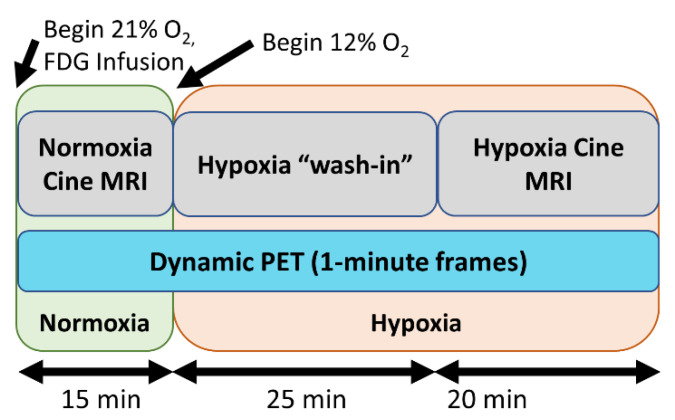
Imaging protocol: In the first 15 min, the subjects breathe 21% oxygen. Subsequently, the oxygen levels are reduced to 12% for 45 min. Cine MRI data are acaquired during the normoxia and, after a 25 min period of a hypoxia ‘washin’ phase, during hypoxia. (18)F-fluorodeoxyglucose (FDG) is continuously infused and dynamic PET data are continuously acquired throughout the imaging exam.
